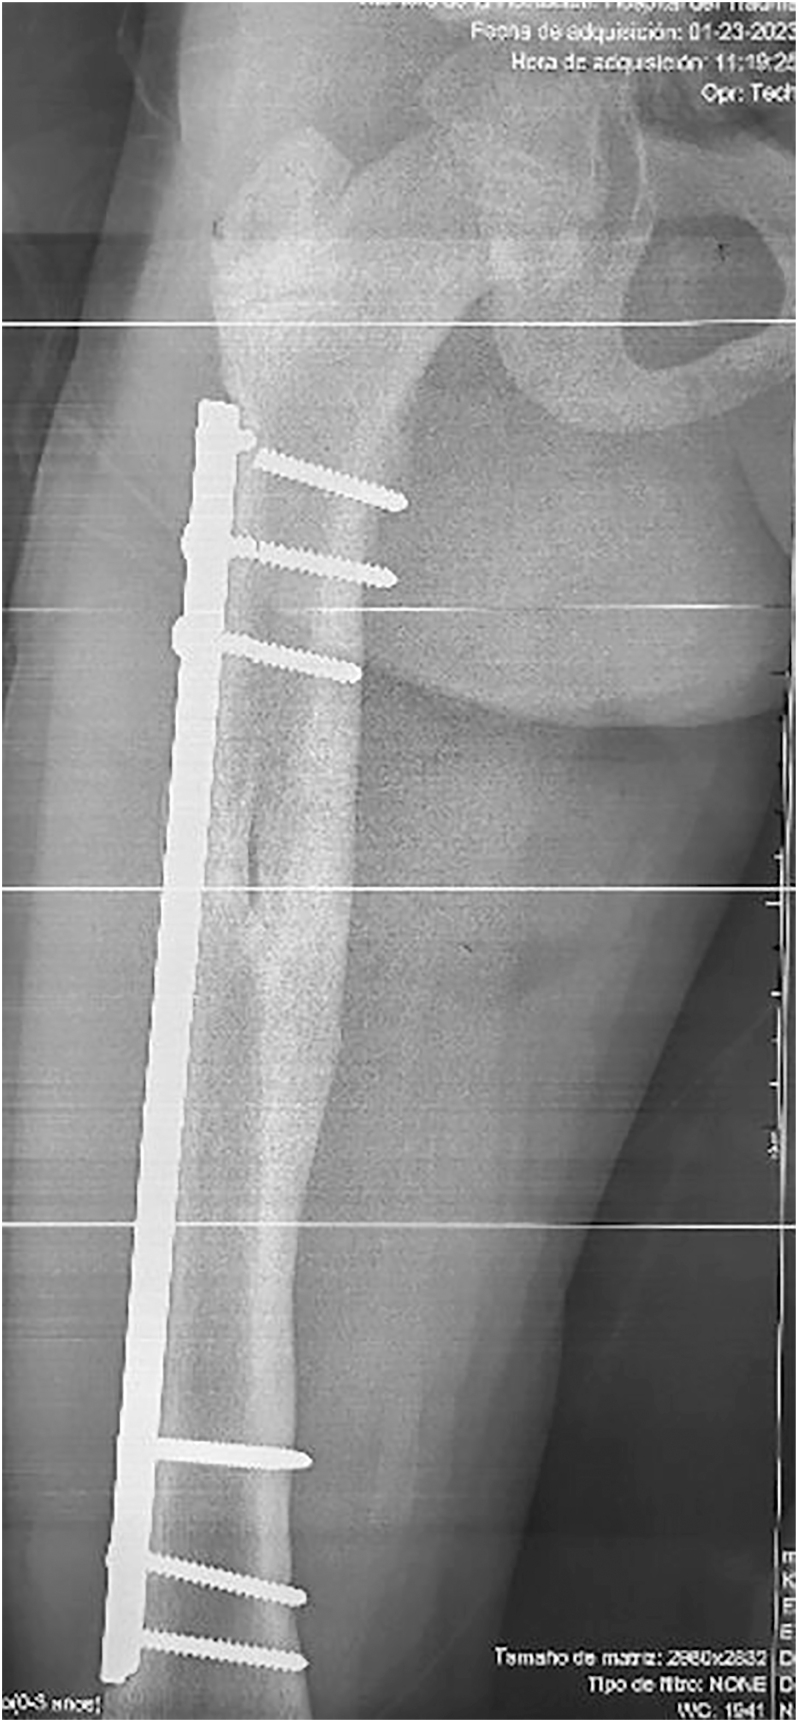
Anteroposterior radiograph showing implant failure after 12 months *in situ* due to breakage of the three proximal screws.
